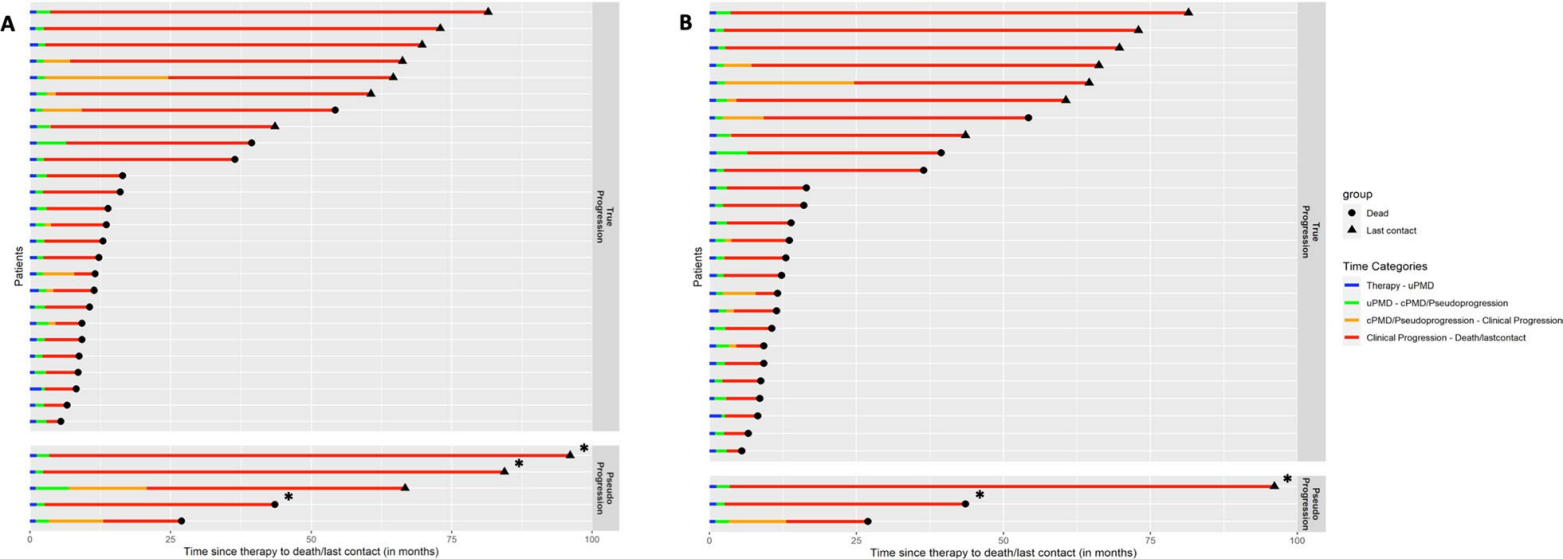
Plots of events of the studied patient cohort during the follow-up period after application of EORTC (*n* = 31 patients) (a) and PERCIMT (*n* = 29 patients) (b). The events depicted are: date of therapy commencement, date of uPMD, date of cPMD or remission of pseudoprogression, date of clinical progression, and date of death or last contact. For both sets of criteria, patients were dichotomized in those showing true progression/cPMD (upper part of the figures), and those showing pseudoprogression (lower part of the figures). *These patients did not show clinical disease progression according to the evaluation of the dermato-oncologists. In these cases, the red part of the lines of events corresponds to the time interval between remission of pseudoprogression in PET/CT and date of death or last contact
